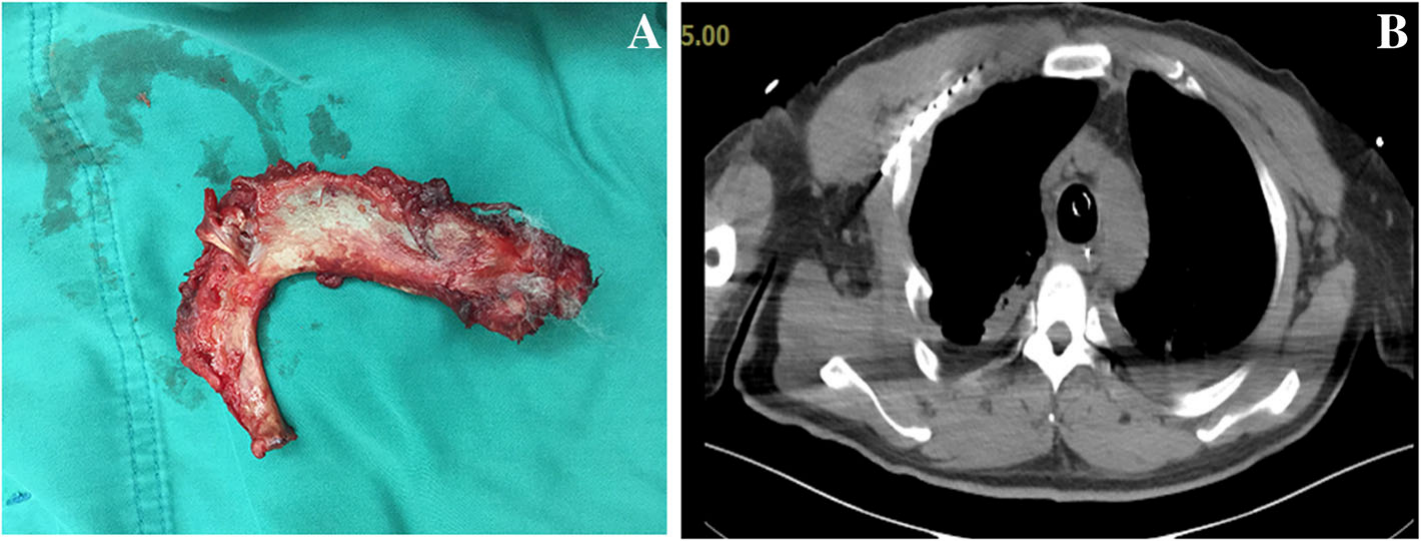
a The right first rib was removed. b Postoperative chest CT-scan showing the integrity of posterior tracheal wall
